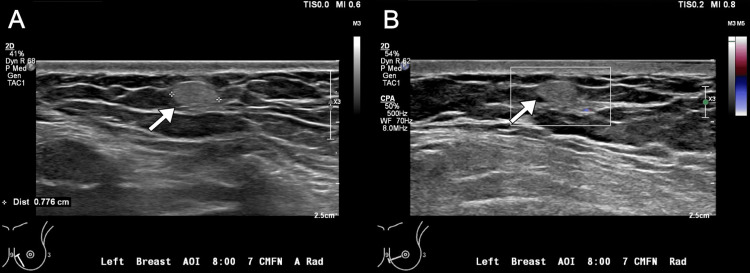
Diagnostic sonographic images of the left breast. (A) Grayscale and (B) color Doppler images demonstrate an 8-mm circumscribed, oval, homogeneously hyperechoic mass (arrows) without associated vascularity superficially in the lower inner quadrant of the breast, 7 cm from the nipple.
